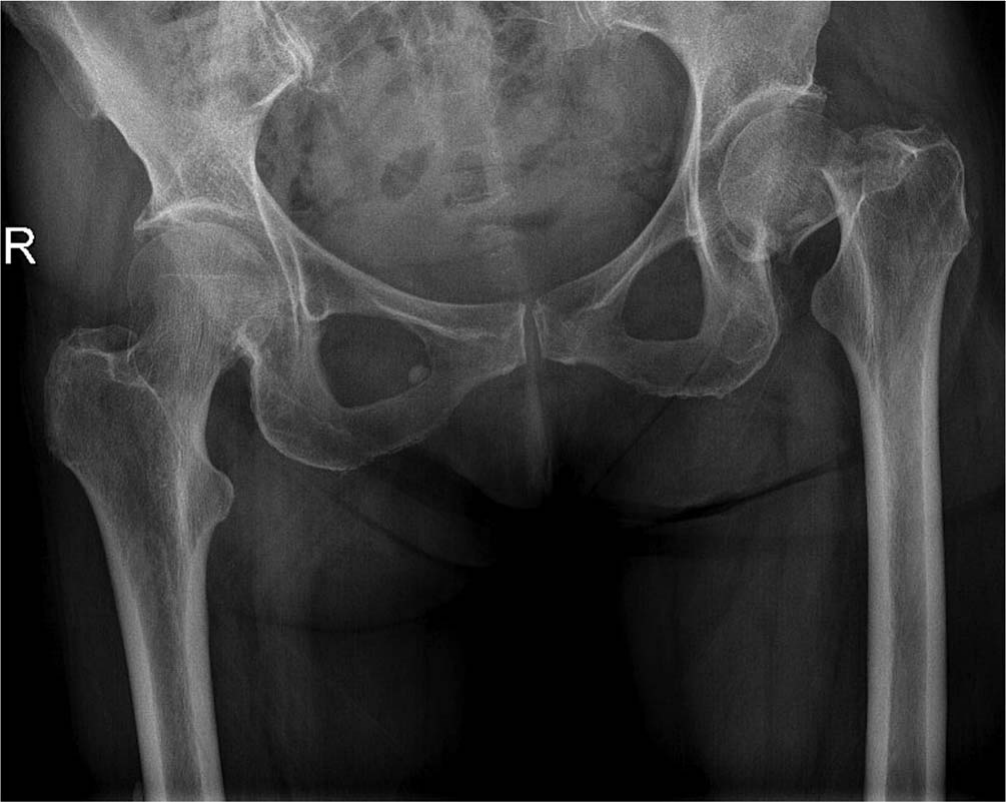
X-ray pelvis showing acute displaced and impacted left femoral transcervical fracture.
